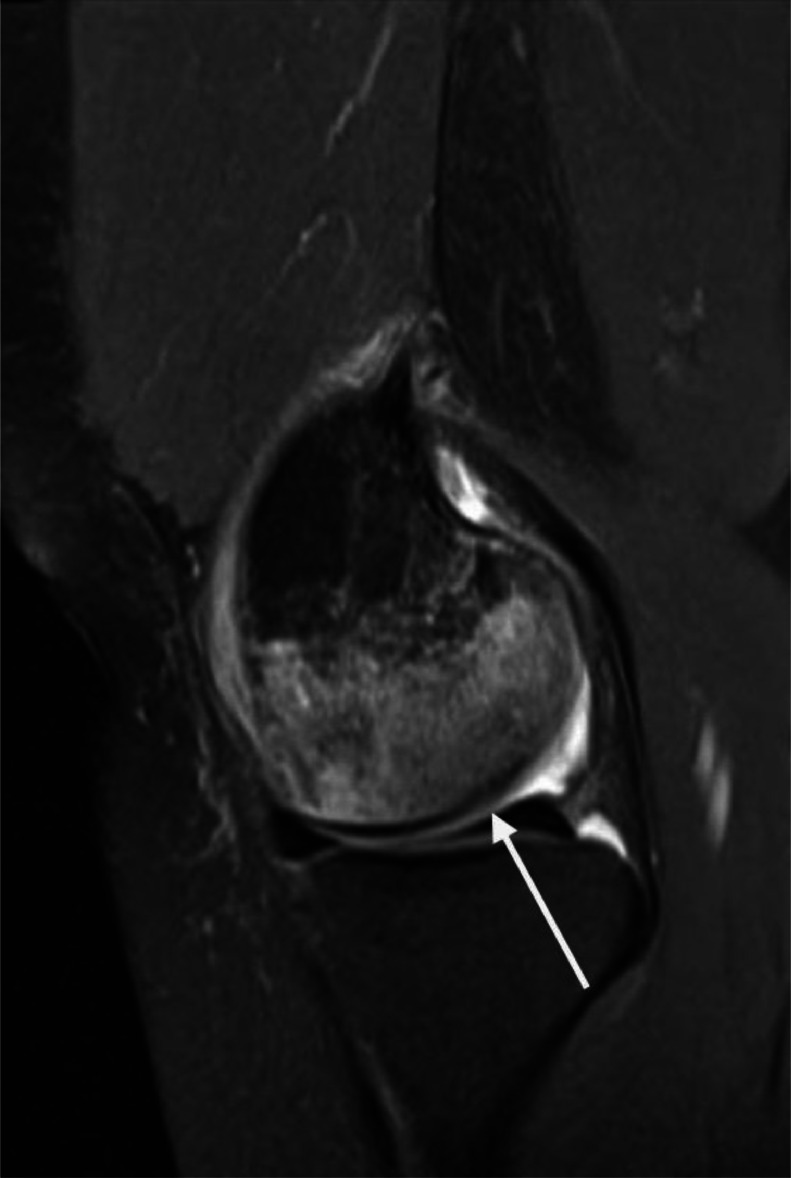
Sagittal PD fluid sensitive MRI sequence. There is a small knee effusion with intact menisci. There is florid high signal within the medial femoral condyle in keeping with extensive marrow oedema. There is irregularity of the subchondral bone plate.
